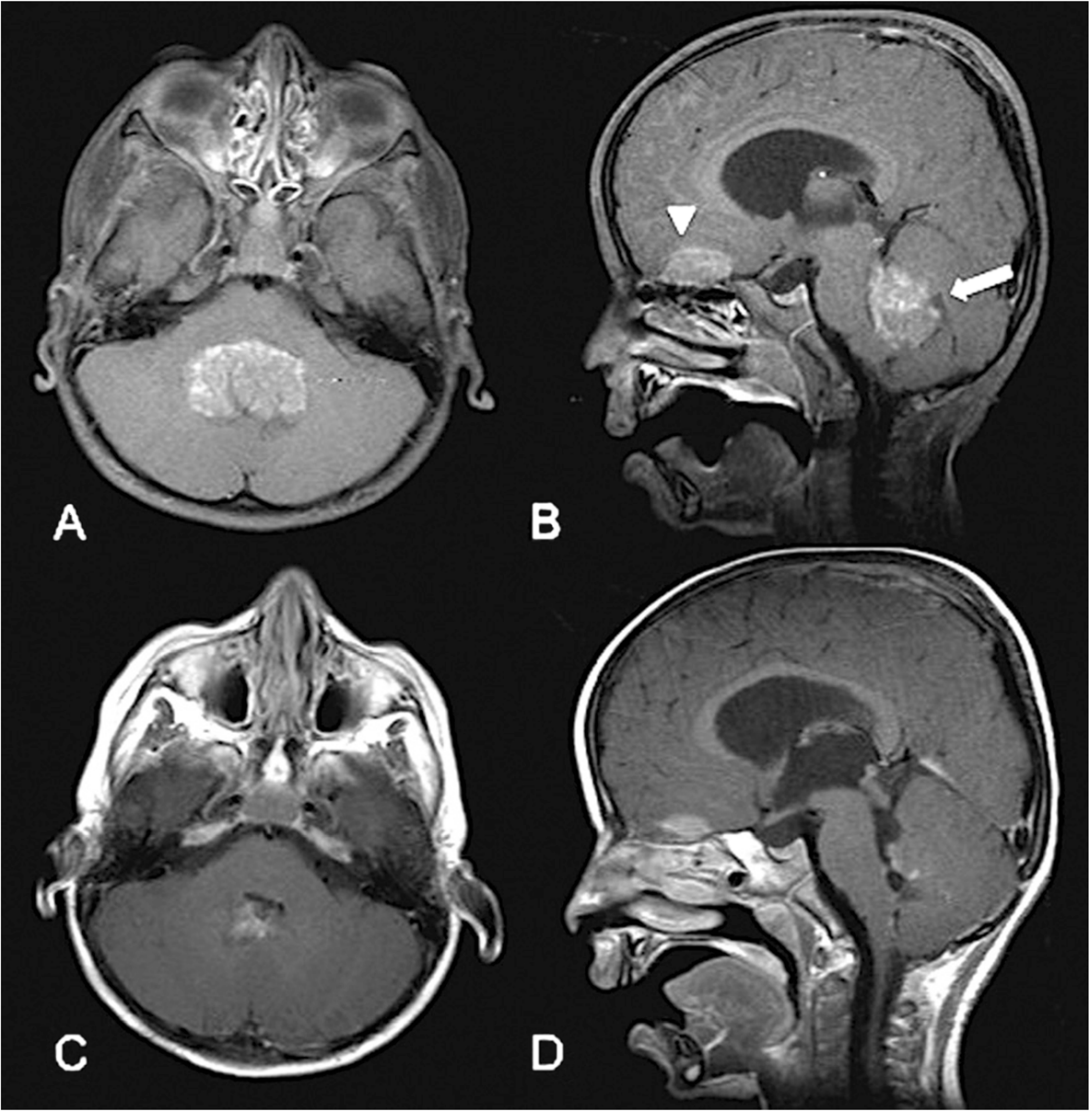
(A) Axial magnetic resonance imaging T1-weighted image with gadolinium, showing a posterior fossa tumor. (B) Sagittal magnetic resonance imaging T1-weighted image with gadolinium showing posterior fossa tumor (arrow) and frontobasal tumor (arrowhead), suggestive of cerebrospinal fluid (CSF) metastasis. (C,D) Post-treatment, one-year follow-up axial and sagittal magnetic resonance imaging revealed regression of the disease.
